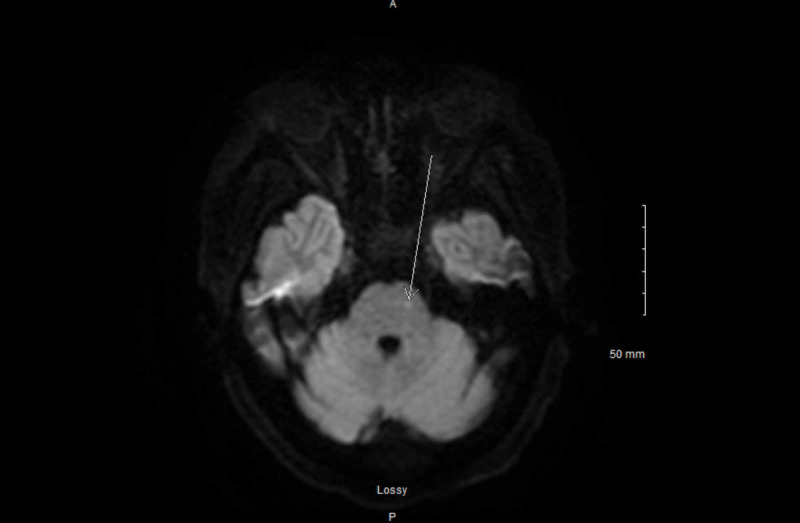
Brain MRI: diffusion-weighted imaging The image shows left pons punctate focus MRI: magnetic resonance imaging
